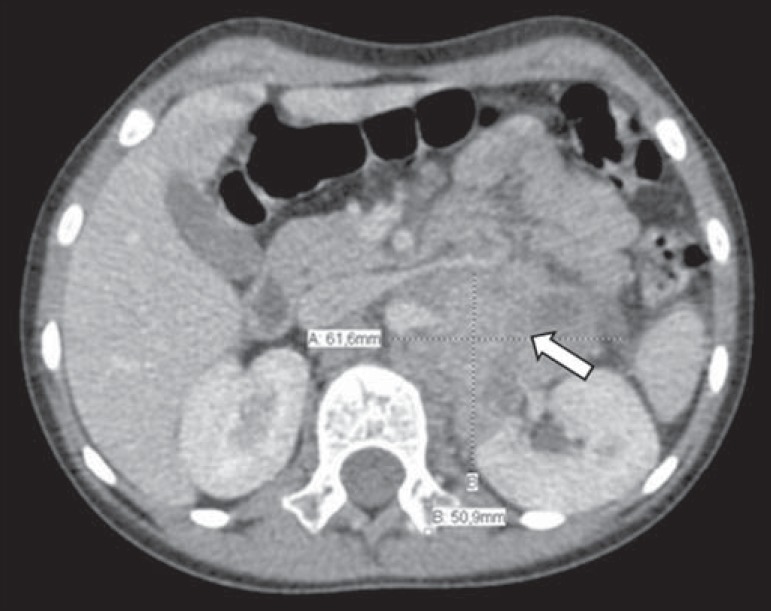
Axial CT image with intravenous contrast showing the anterior
